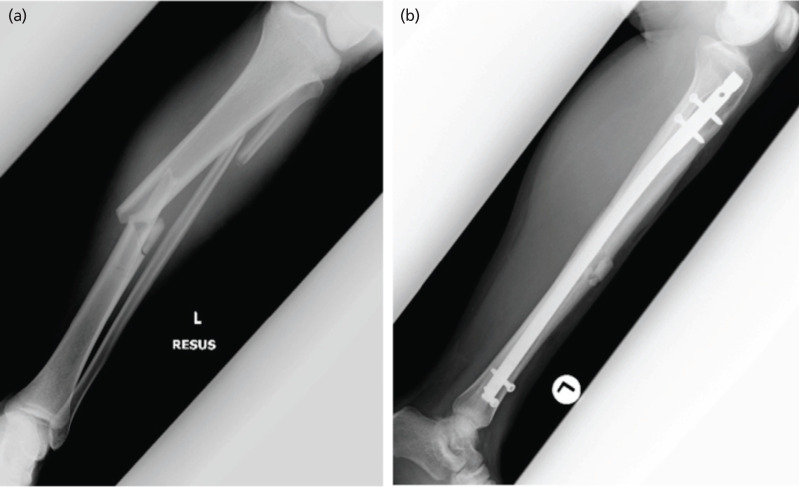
Radiograph images of Case 1. (a) Initial injury on arrival. (b) Post-operative follow-up.
